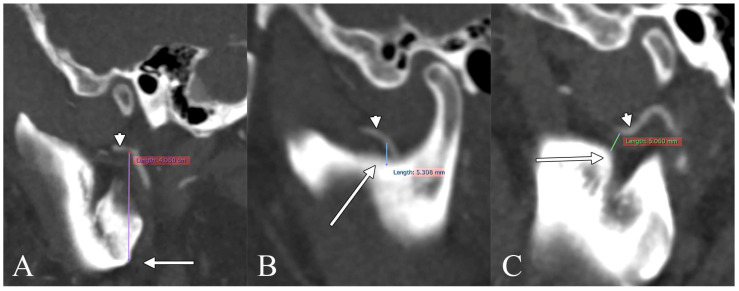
Measurements of distances of the maxillary artery (MA, arrowheads) to specific landmarks on sagittal CTA slices through the mandibular ramus, left side, lateral views (single case). (A) MA–gonial angle (arrow) distance. (B) MA–mandibular notch (arrow) distance. (C) MA–lingula (arrow) distance.
